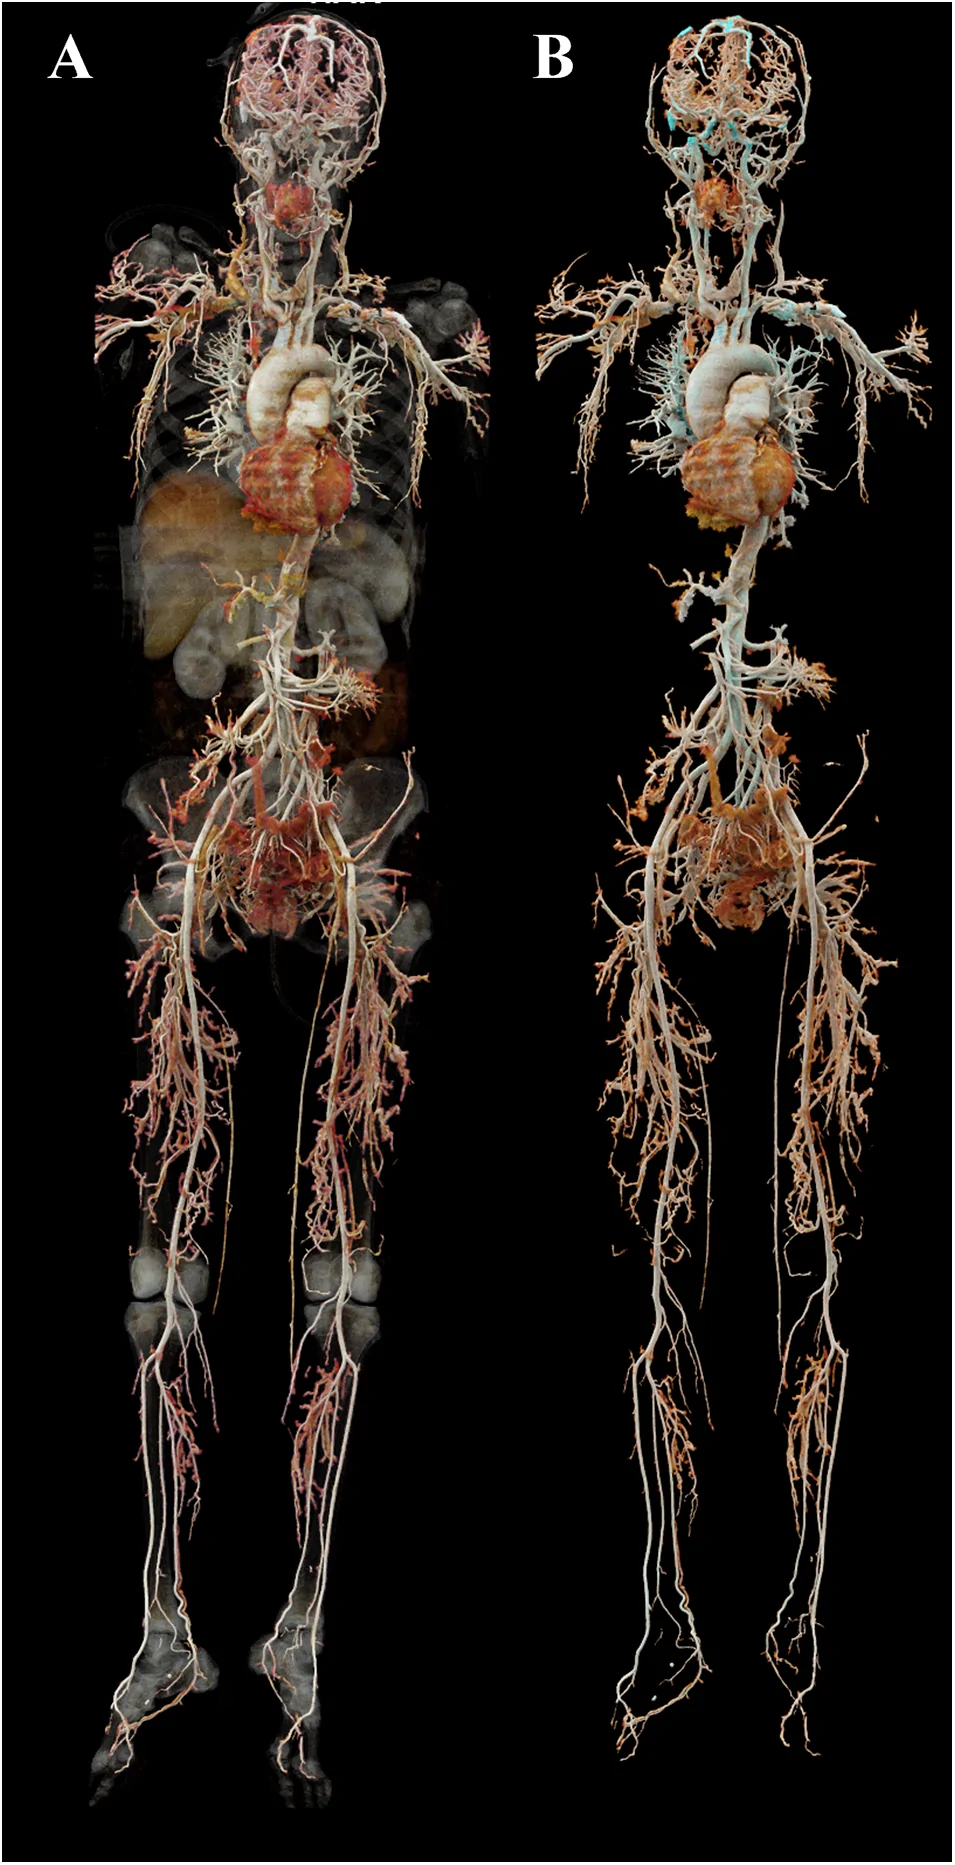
Whole-body vascular imaging of the trauma patient using photon-counting CT angiography reconstructed with cinematic rendering. The image provides a photorealistic three-dimensional overview of the cerebral, cervical, thoracic, abdominal, and lower-extremity arterial trees in a single view, allowing preoperative planning of the pedicled fasciocutaneous flap. (A) Anterior and (B) posterior views. Scale bar: 5 cm.
